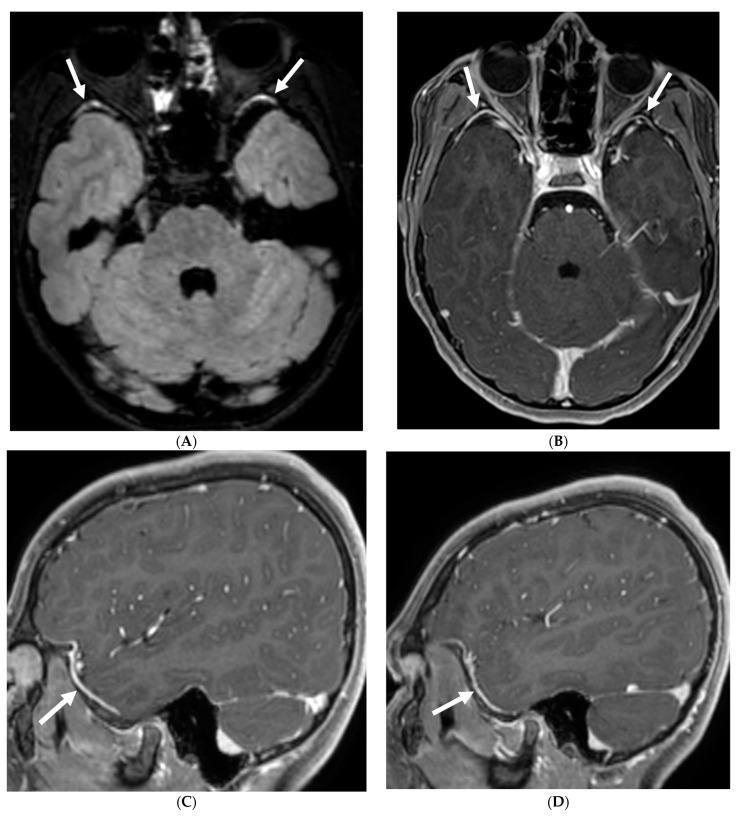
Post contrast axial FLAIR (A), axial T1 FS (B), sagittal T1 Right (C) and Left (D): 10-year-old girl with elevated ANCA, headache and mild LUE weakness. There is bilateral anterior temporal smooth dural enhancement (white arrows). Diagnosis: Antineutrophilic cytoplasmic antibody (ANCA) associated vasculitis, likely granulomatosis with polyangiitis.
